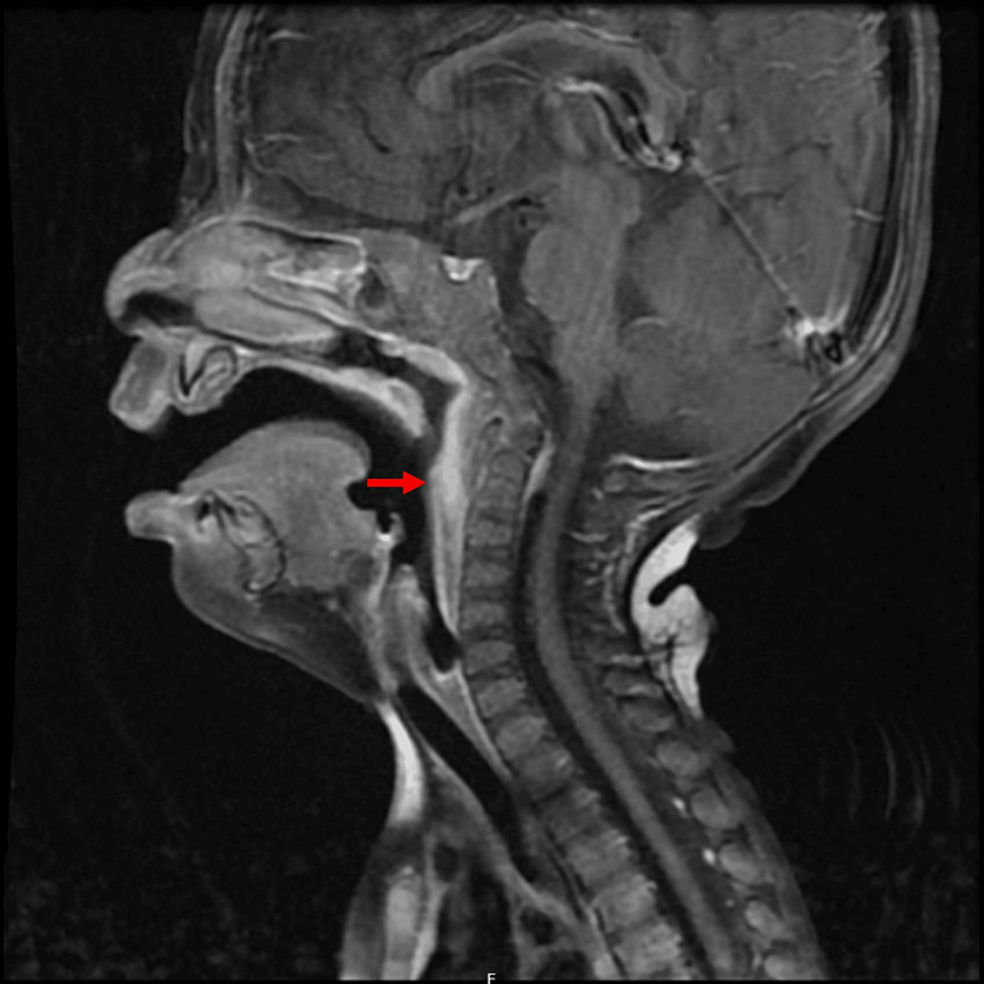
Magnetic resonance imaging (MRI) T1 with contrast coronal view, showing diffuse thickening, edema, and prominent enhancement of the retropharyngeal and prevertebral soft tissues with associated edema.
